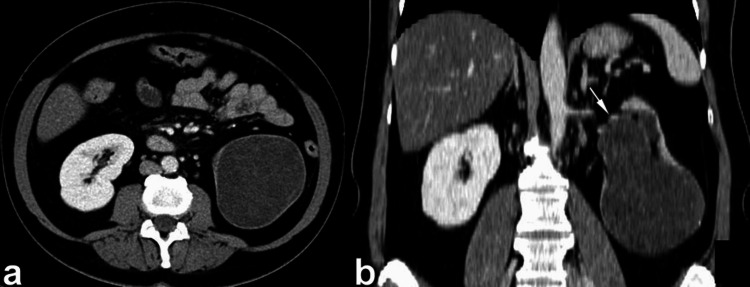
Axial picture (a) and the reconstruction in the frontal plane (b) of a contrast-enhanced CT (CECT) scan during the nephrographic phase unveil a notable pathological finding within the left kidney. Specifically, a multiloculated cystic mass is prominently displayed, indicating the presence of multiple septa. This mass is encapsulated by a robust fibrous capsule, suggestive of a well-defined boundary separating it from the surrounding renal parenchyma. Notably, the presence of this thick fibrous capsule exerts pressure on the adjacent renal parenchyma with cortical thinning. Furthermore, the capsule extends into the renal sinus, as indicated by the arrow, underscoring the invasive nature of the lesion. This figure is a republished image, permission was obtained from the original publisher, https://epos.myesr.org/poster/esr/ecr2018/C-2802
